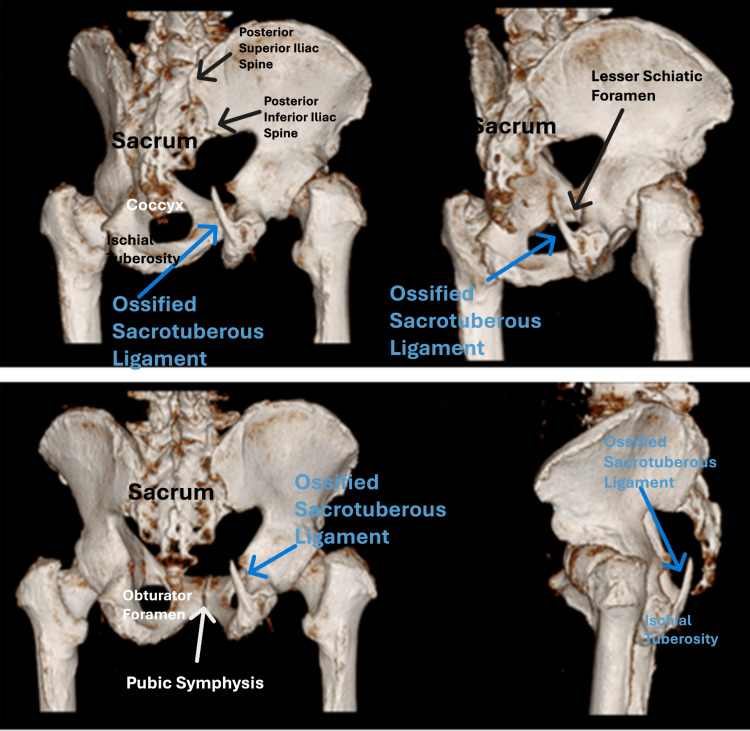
Three-dimensional computed tomography (3D-CT) reconstructions of the pelvis demonstrating partial ossification of the right sacrotuberous ligament The ossified ligament appears as an elongated, fan-shaped calcified structure (arrows) extending from the posterior pelvis toward the ischial region, in close anatomical relation to the expected course of the pudendal nerve. Key anatomical landmarks related to the attachment and course of the ossified sacrotuberous ligament are demonstrated, including the sacrum, posterior superior iliac spine, posterior inferior iliac spine, coccyx, ischial tuberosity, lesser sciatic foramen, obturator foramen, and pubic symphysis.
